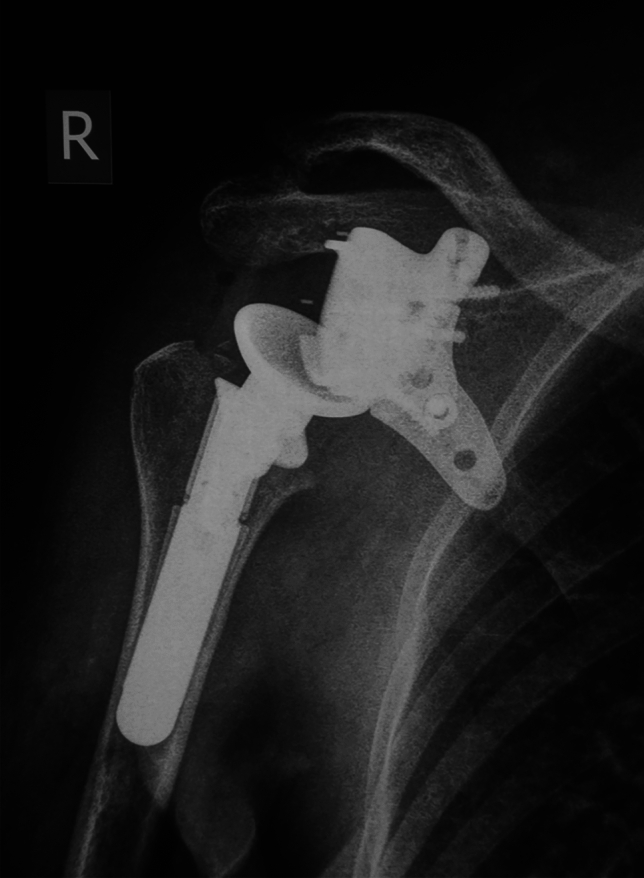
Plain radiograph of the right shoulder 28 months postoperatively
